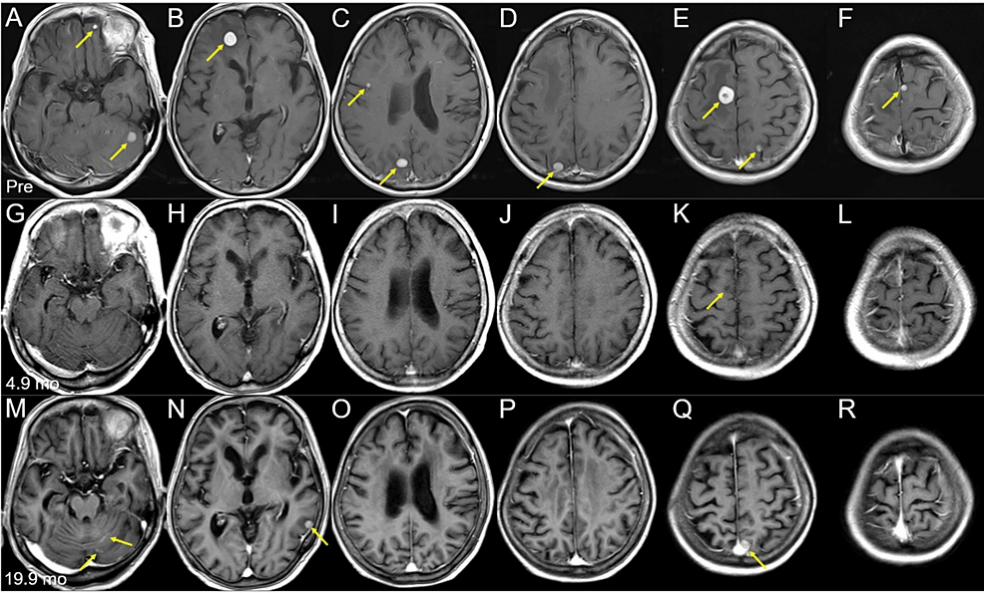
Brain magnetic resonance images of the other representative lesions at the initial diagnosis and after whole-brain radiotherapy The images show axial CE-T1-WIs (A-L); before (pre) the initiation of WBRT (A-F); at 4.9 months (mo) after WBRT initiation (G-L); and at 19.9 months (M-R). (A–R) These images are shown at the same magnification and coordinates under co-registration and fusions. (A-F) Nine out of the 15 lesions are visible as solid enhancing masses (arrows in A–F). (G-L) All nine lesions regressed completely, only leaving the cavitary scar in the right frontal lesion (arrow in K). (M-R) None of the nine lesions with complete responses showed local progression; however, multiple new lesions (arrows in N, Q) appeared, along with localized leptomeningeal dissemination (arrows in M). CE: contrast-enhanced; WIs: weighted images; WBRT: whole-brain radiotherapy
